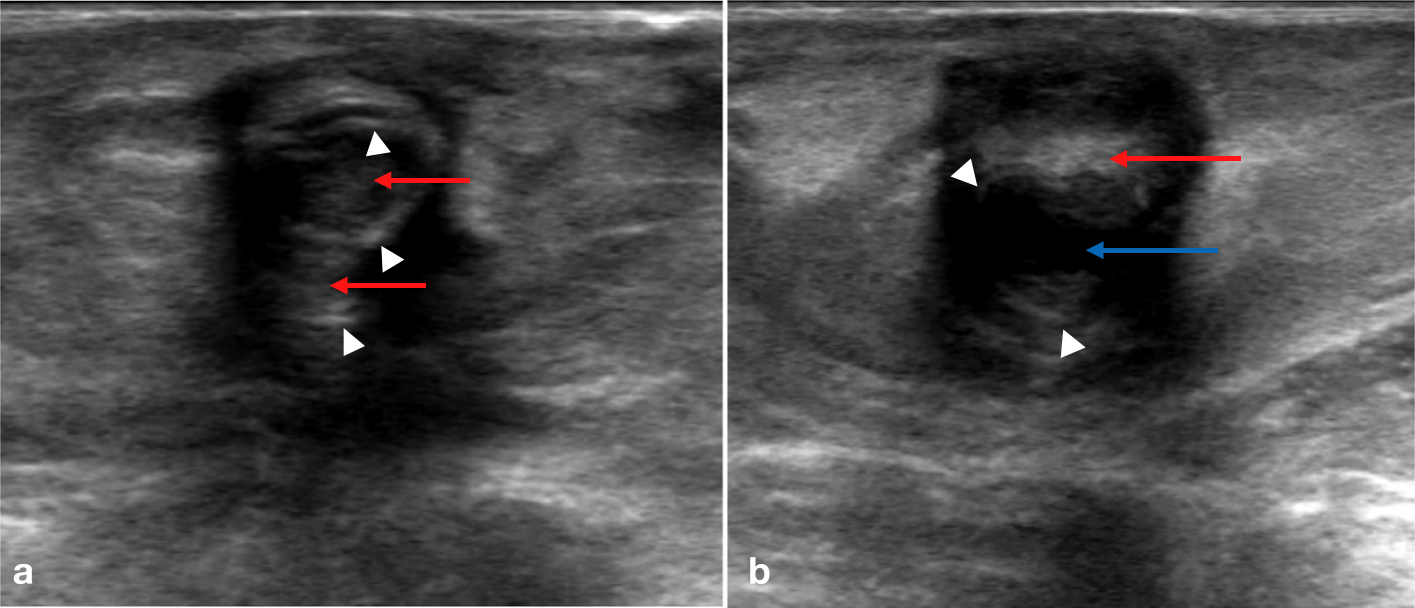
a, b Doppler ultrasonography of left brachio-axillary arteriovenous graft showing echogenic linear material floating distinctly within the graft lumen (blue arrow), suggestive of delaminated graft fabric layers (arrowheads). It also shows thrombus in the false lumen created by graft delamination and an intraluminal thrombus (red arrows) (a)
